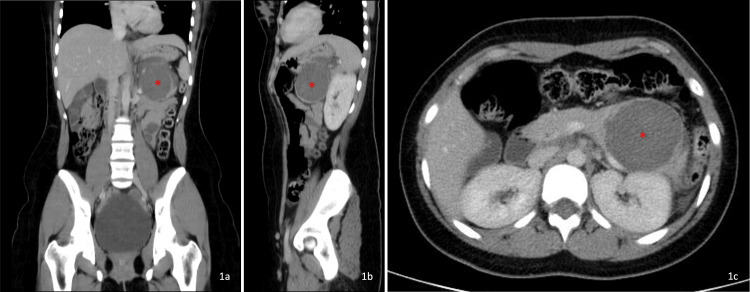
Abdominal and pelvic computed tomography (CT) with an abdominal window A well-defined cystic lesion was identified in the tail of the pancreas (red "*"), visualized on coronal (Figure 1a), sagittal (Figure 1b), and axial (Figure 1c) planes on contrast-enhanced CT in the venous phase. It demonstrated homogeneous low attenuation after contrast administration, without an apparent solid component (27 Hounsfield units); an unenhanced phase was not performed. The lesion measured approximately 68.1 × 56 mm in its largest dimensions. A small amount of free fluid was noted in the left paracolic gutter (Figure 1c). No other significant findings were identified.
